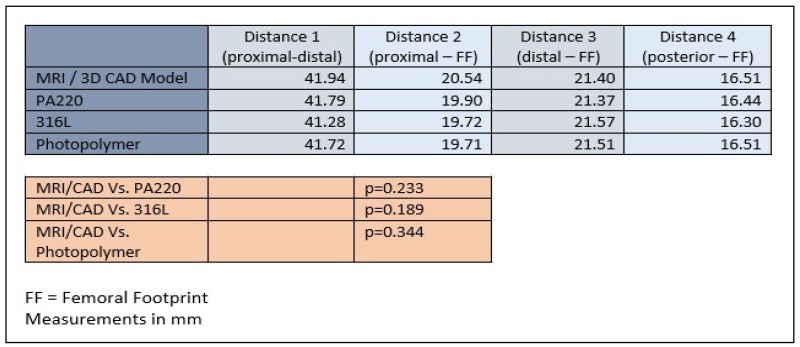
Mean measurements of patient specific ACL femoral tunnel guides. Distances measured included proximal to distal articular cartilage (distance 1), posterior articular cartilage to femoral footprint (distance 2), distal articular cartilage to femoral footprint (distance 3) and proximal articular cartilage to femoral footprint (distance 4). No statistical significant differences were found in the size of models or the position of the femoral footprint when compared to the computer assisted design or patient MRI. FF = Femoral Footprint. Measurements in mm.
